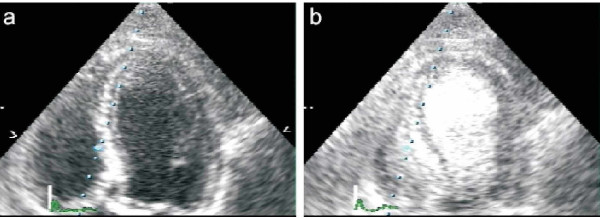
Apical 4 chamber view with LK565 Apical 4 chamber view before (a) and 1 minute after (b) intravenous LK565 application (4 mg/kg BW). Device: SONOS 5500, S3 Probe, harmonic
